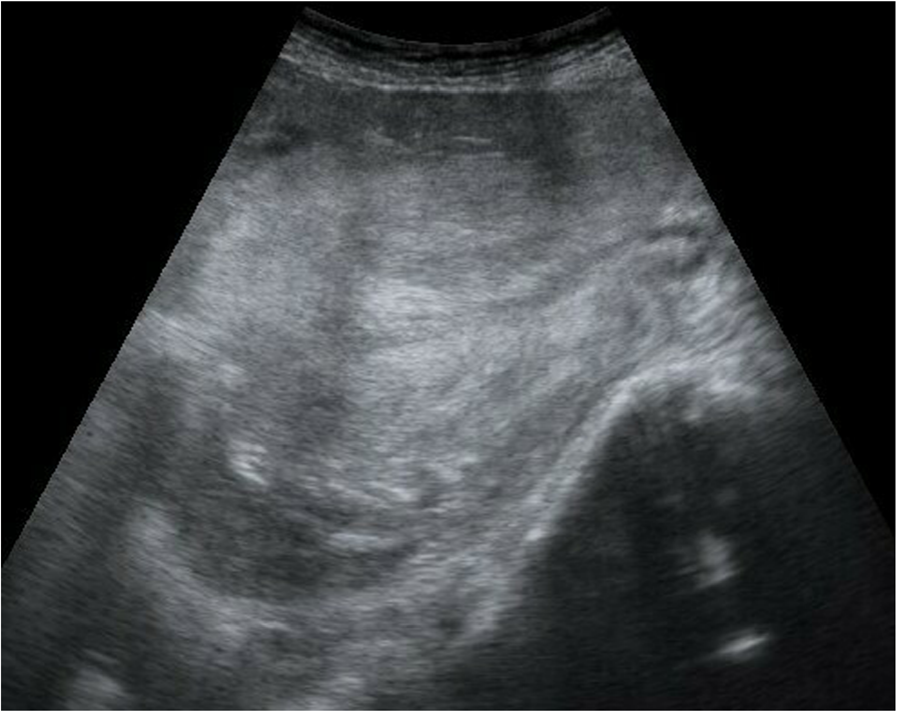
Abdominal ultrasonography findings. The uterine fundus passes through the cervical ring (sagittal view: right side – patient’s head, left side – patient’s leg)
